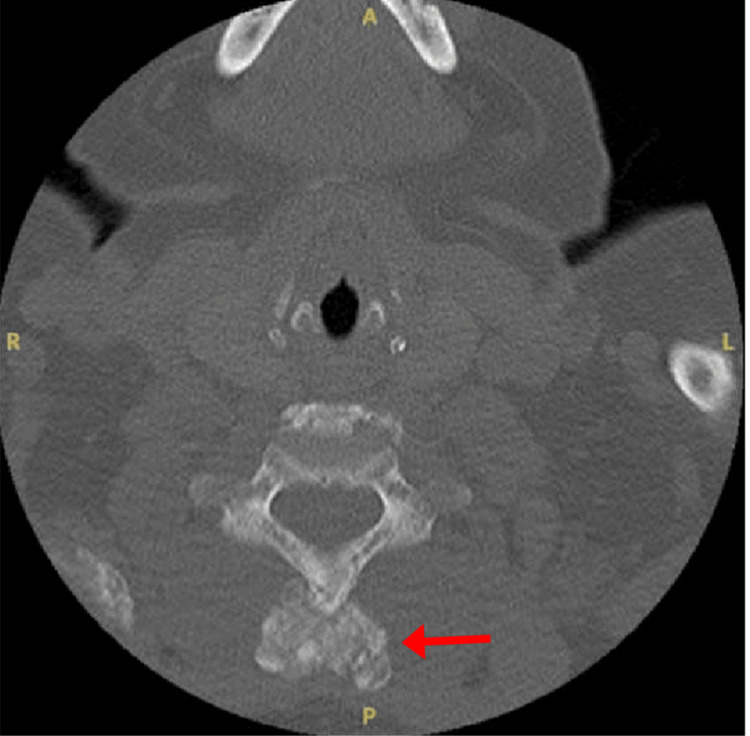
CT axial view demonstrating spinous process involvement.
